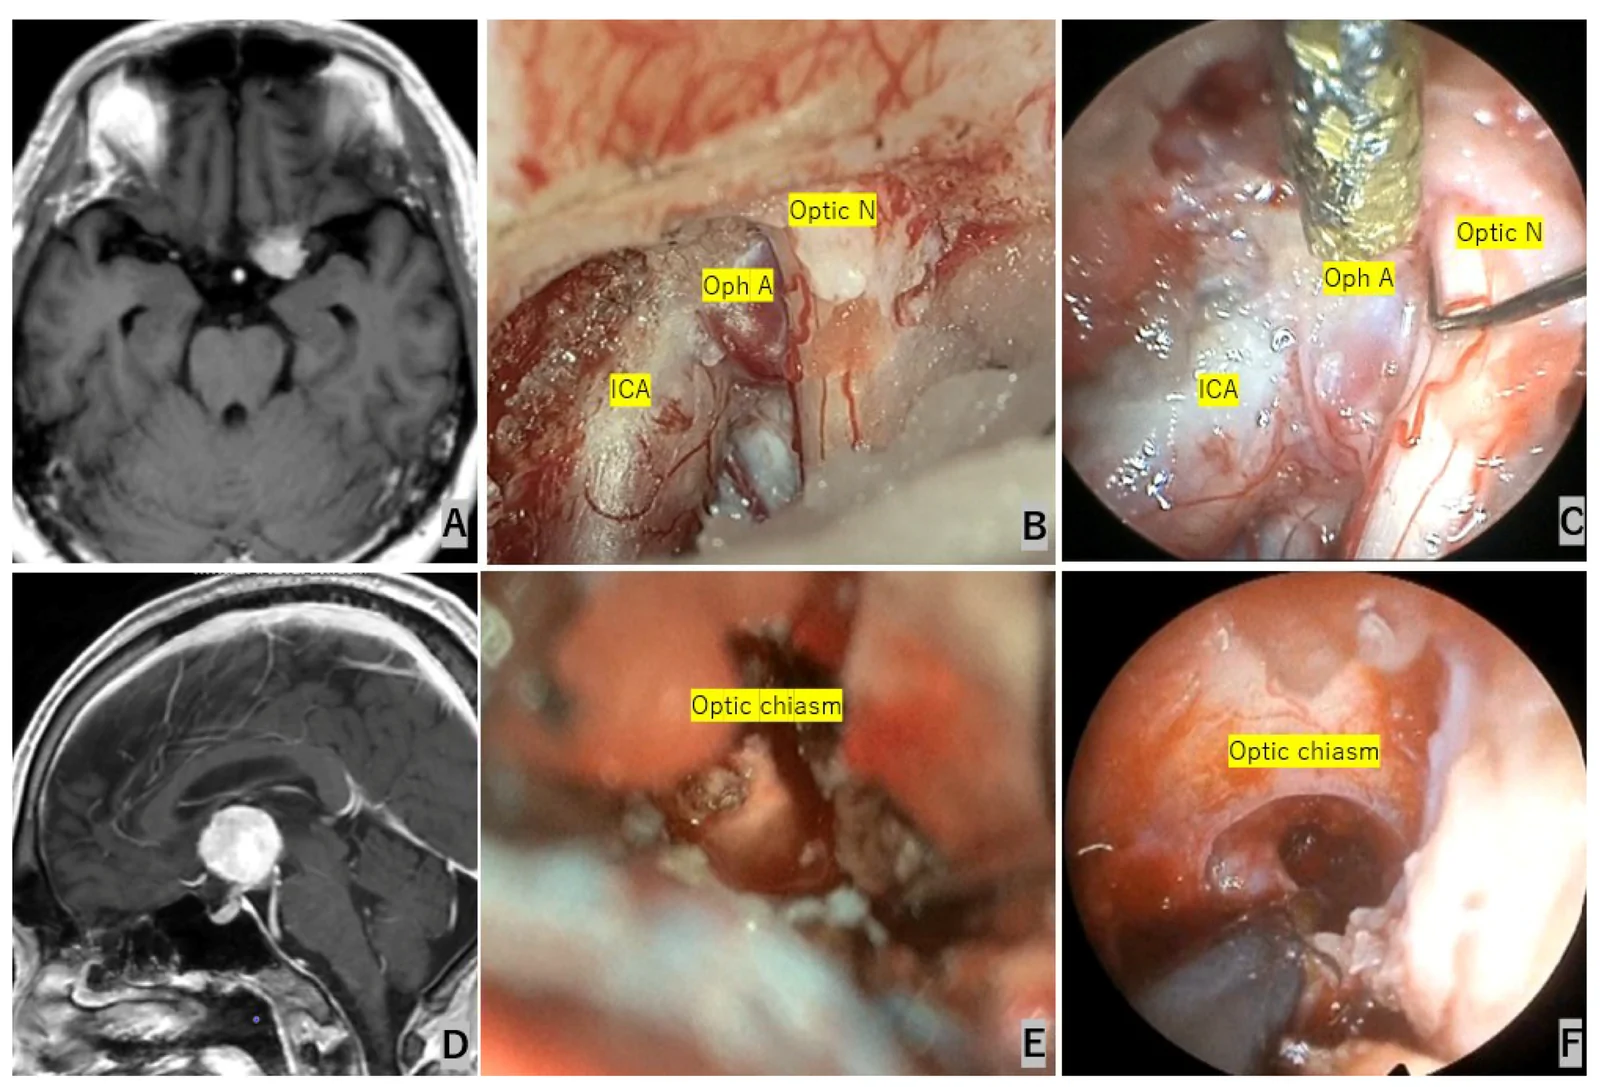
Representative applications of neuroendoscopic assistance during optic canal inspection and simultaneous transcranial-endonasal surgery. (A–C) Inspection of the optic canal during resection of a medial sphenoid wing meningioma. (A) Preoperative contrast-enhanced magnetic resonance imaging demonstrating tumor extension toward the optic canal. (B) Exoscopic intraoperative view. (C) Endoscopic view providing enhanced visualization of the optic canal and its contents. (D–F) Simultaneous transcranial and endonasal surgery for a chordoid glioma. (D) Preoperative contrast-enhanced magnetic resonance imaging demonstrating a third ventricular chordoid glioma. (E) Exoscopic view obtained through the transcranial corridor. (F) Endoscopic view obtained through the endonasal corridor, allowing real-time integration of information from both surgical trajectories. Oph A, ophthalmic artery; Optic N, optic nerve.
